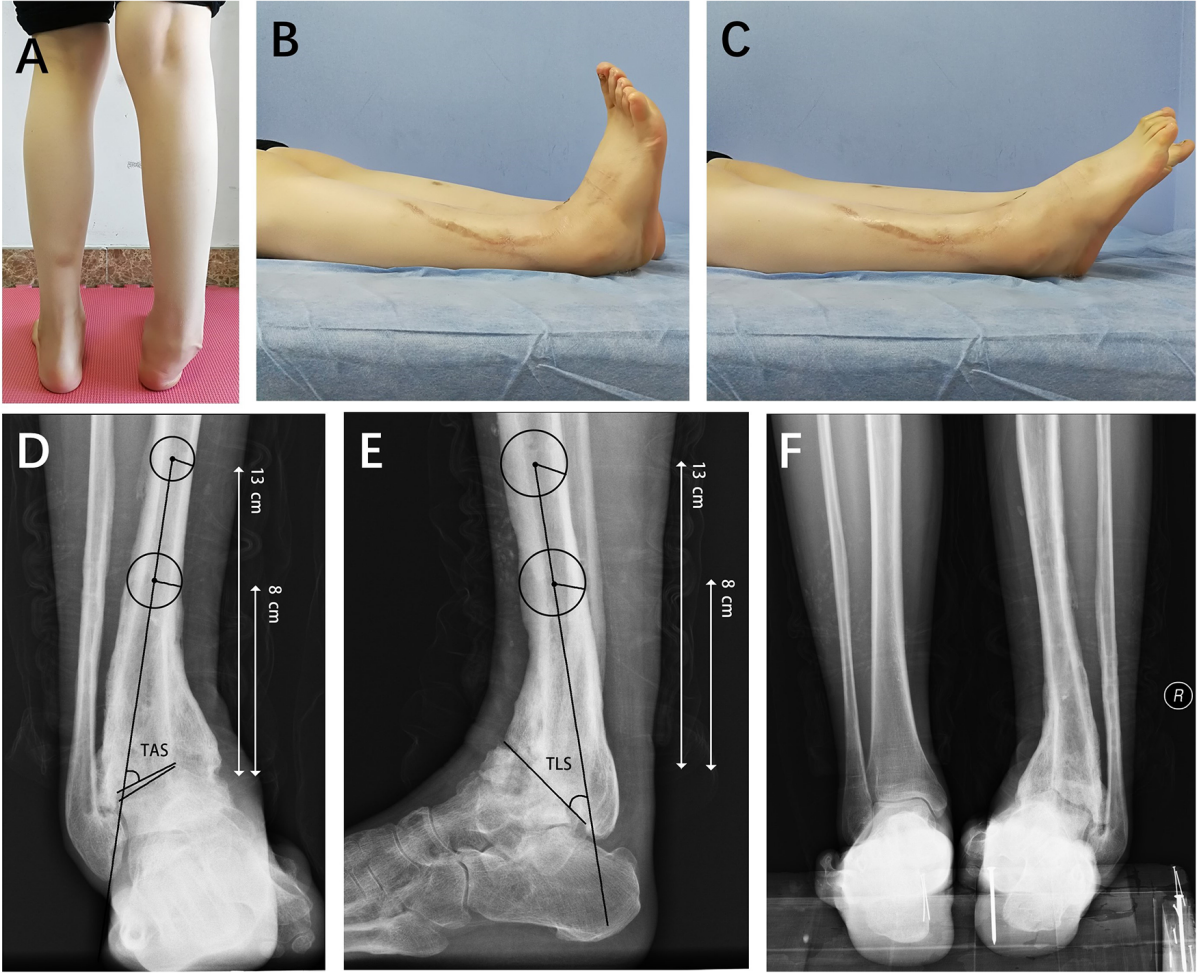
The internal fixation devices were removed 18 months after the initial surgery. The deformity of the right ankle is shown in panel (A), and the range of motion is displayed in panel (B,C). Radiographic measurements revealed that the angles of the tibial articular surface (TAS) and tibial lateral surface (TLS) were 54 and 50 degrees, respectively (D,E). Panel (F) depicts the hindfoot malalignment.
